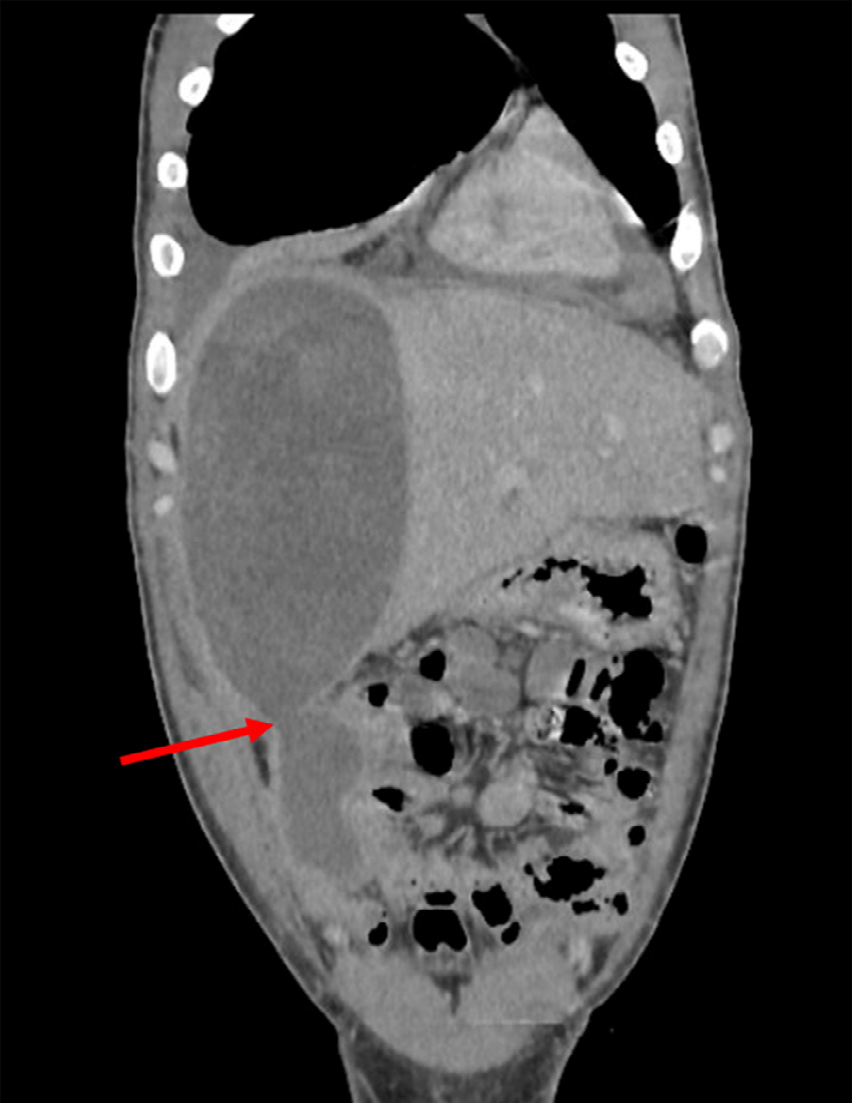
A coronal slice of a CT scan showing the rupture of the huge right hydatid cyst in the peritoneal cavity (red arrow).
